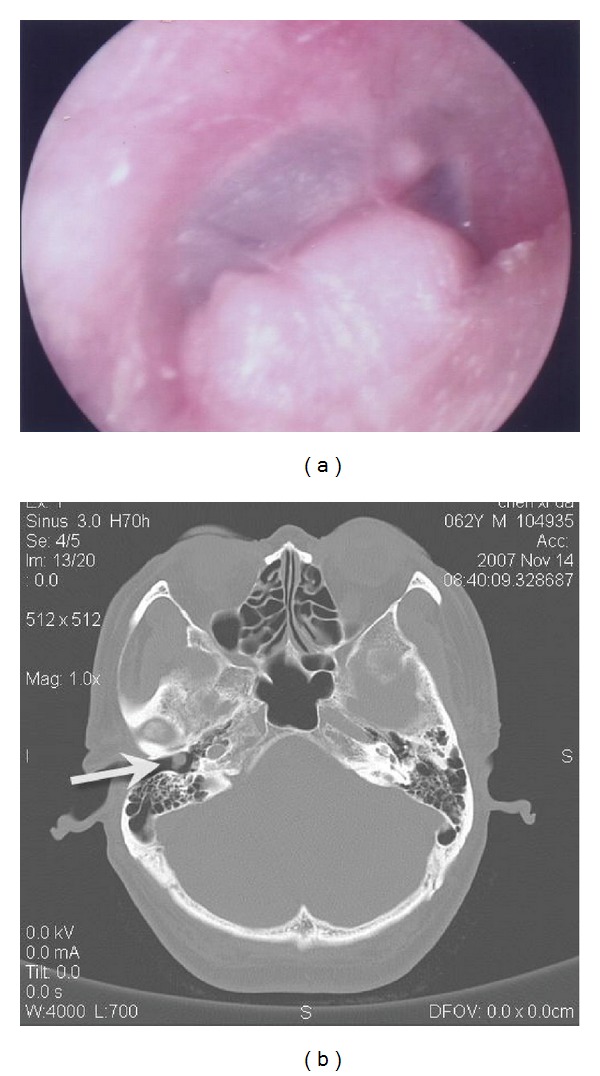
Auxiliary examinations including (a) myringoscope which showed a flesh-colored mass in the anterior and inferior walls of deep right external auditory canal, near the tympanic membrane. (b) Axial cranial CT which presented a well-circumscribed soft tissue mass with homogeneous density (white arrow) in the right external auditory canal.
